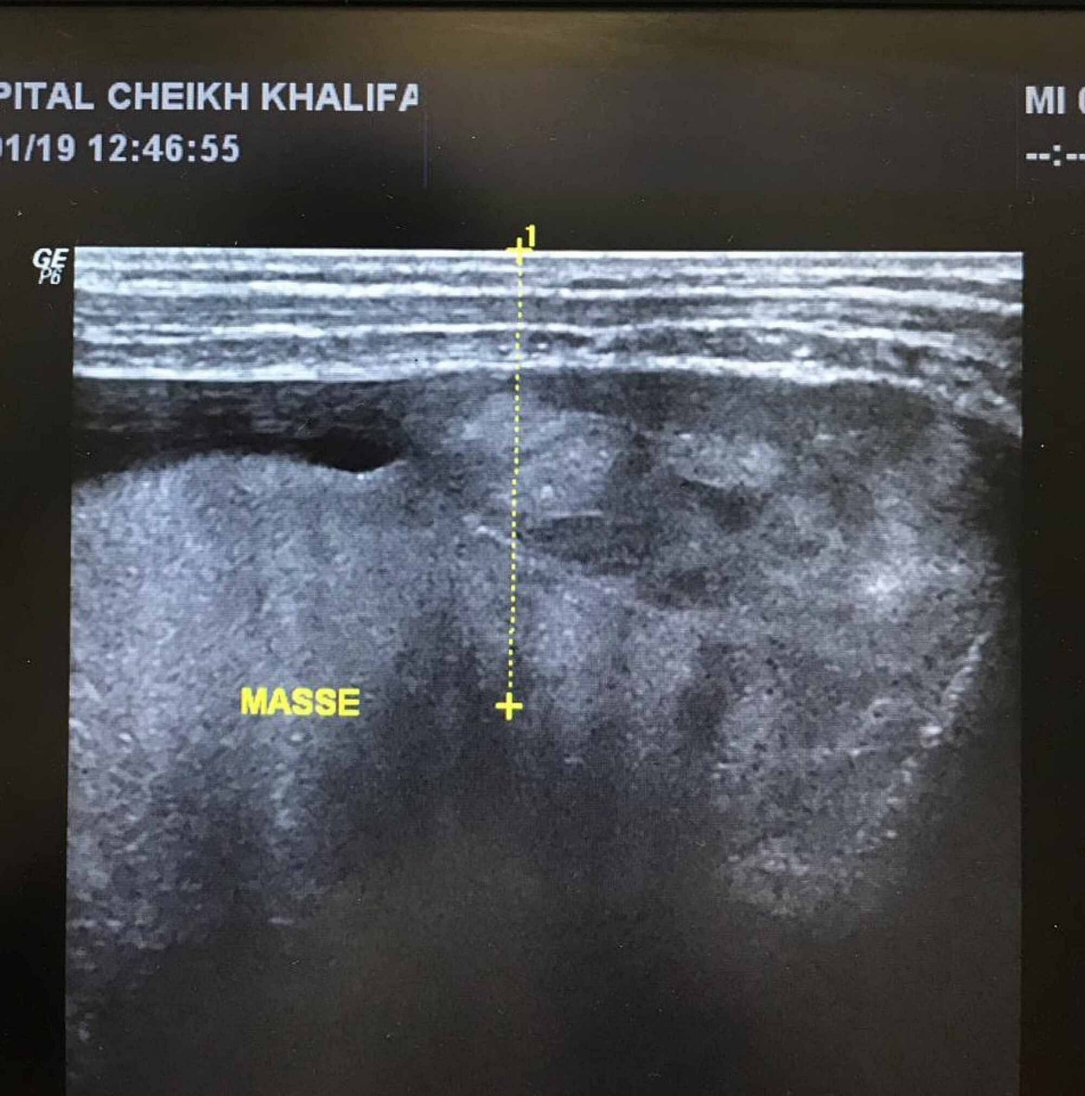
Chest ultrasound showing a multinodular mass and guiding the puncture-biopsy.
